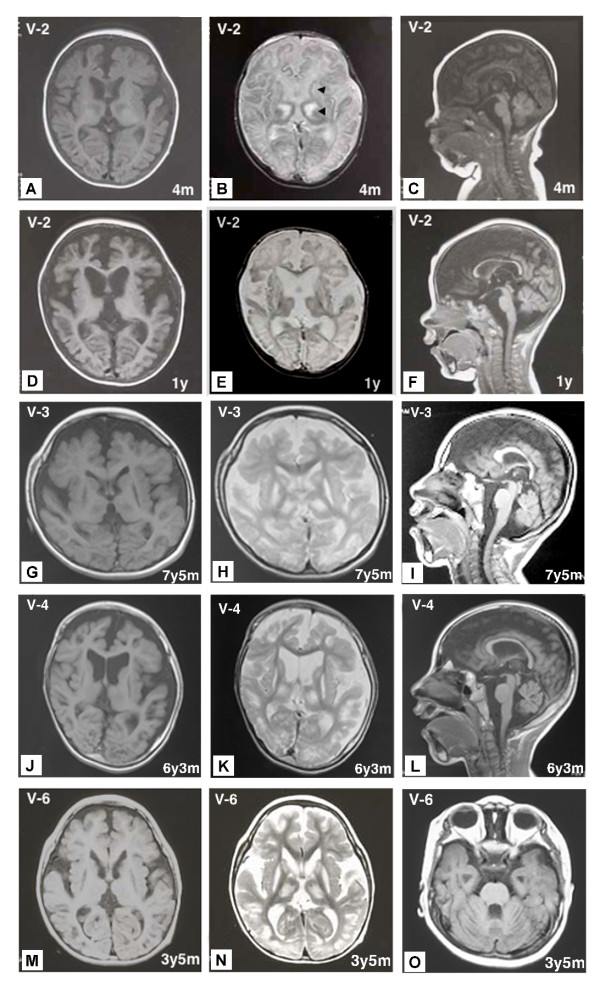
MRIs of patients V-2, V-3, V-4 and V-6 at various ages. Areas of abnormal intensity in the thalami and basal ganglia (arrowheads) were distinct at 4 months in V-2 (A, B). The sagittal and axial views of T1- and T2-weighted images of V-2 at 1 year showed severe brain atrophy (D, E, F). The sagittal and axial views of T1- and T2-weighted images of V-3 at 7 years, 5 months (G, H, I), V-4 at 6 years, 3 months (J, K, L), and V-6 at 3 years, 5 months (M, N, O) showed severe brain atrophy, including cerebellar atrophy (I, L, O) and abnormal intensity areas in the thalami and basal ganglia.
